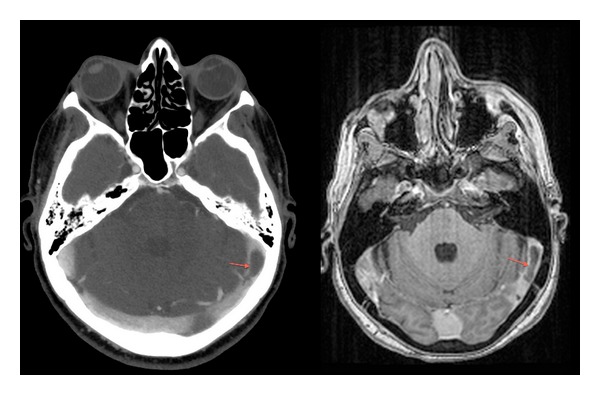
T1-weighted brain magnetic resonance showing left transverse sinus thrombosis (arrows).
